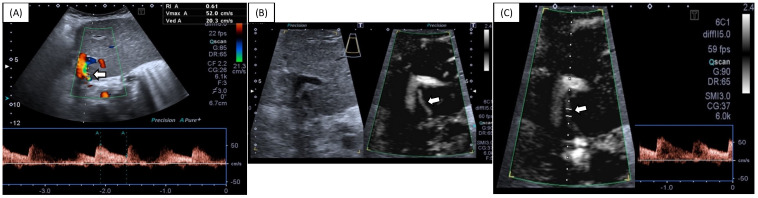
A 10 year old female undergoing standard abdominal US follow-up for prior LT. HA assessment is performed with CDI (A) and SMI (B,C). Visualization and sampling of the HA (arrows) are comparable in this case. Arrows indicate HA. HA—hepatic artery; CDI—conventional Doppler imaging; SMI—superb microvascular imaging.
